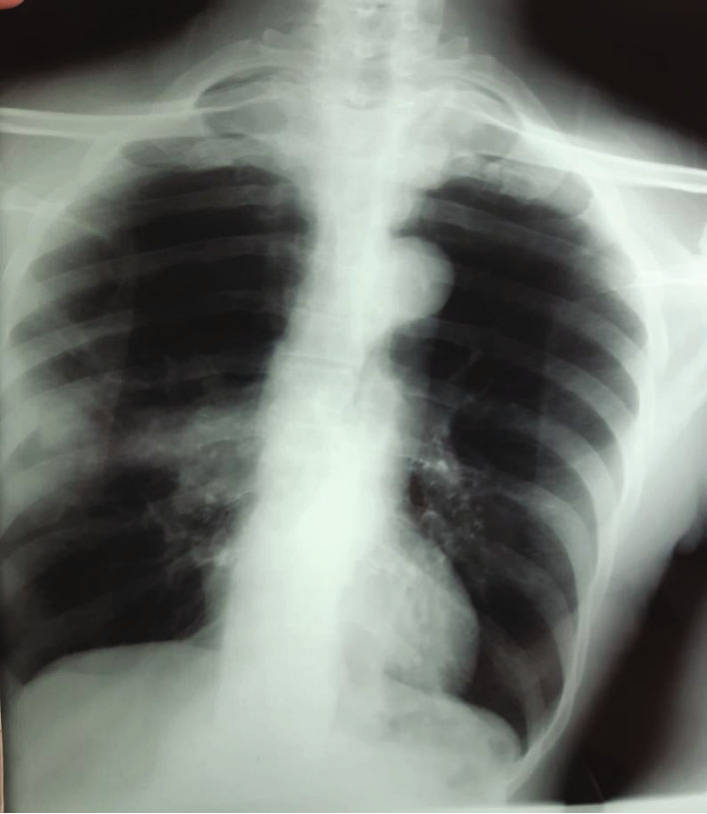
The posteroanterior chest roentgenogram showed homogeneous right hilar opacity with peripheral nodular opacity with spiculated contours.
